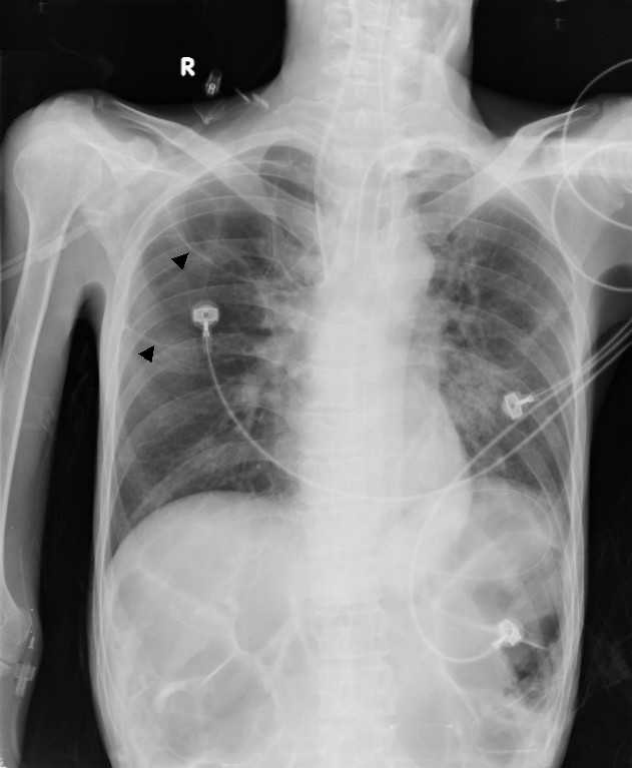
Chest radiograph at 3-month showing right upper lobe opacity replaced by multiple thin-walled cystic lesions (arrowheads) measuring greater than 4 cm in diameter.
